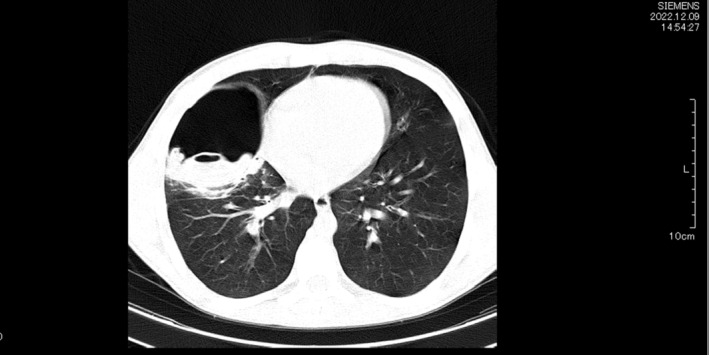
Chest CT scan showing a large cavitary lesion on the middle lobe of the right lung which contains a large amount of air, and soft tissue resembling a membrane (Water Lily sign).
